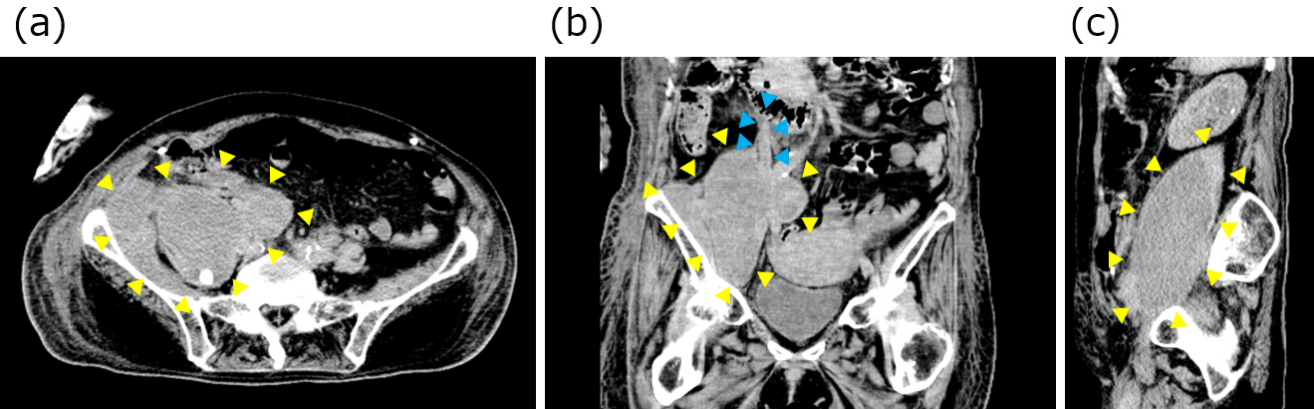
Abdominal CT at admission. The yellow triangular arrow indicates the IPA and the blue triangular arrow indicates the ureter. Communication between the ureter and the iliopsoas abscess was observed. (a) Axial view. (b) Coronal view. (c) Sagittal view.
